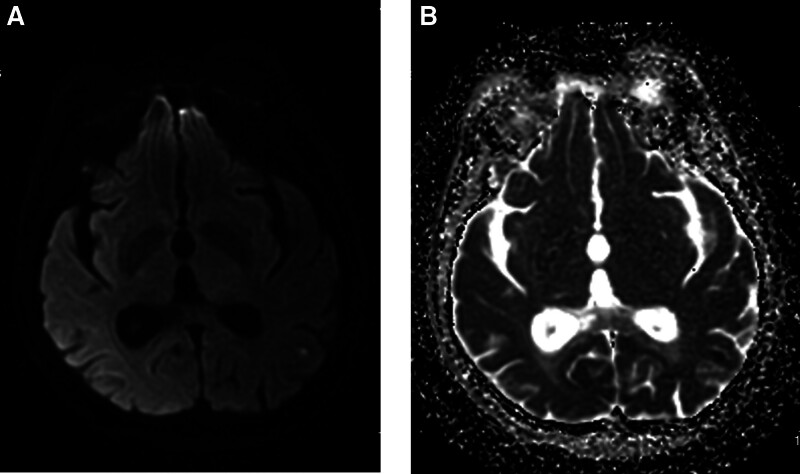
Follow-up cranial MRI after CAS. (A) Magnetic resonance DWI imaging after CAS, DWI point-like high signal in the left parietal lobe, with possible acute/subacute cerebral infarction. (B) Magnetic resonance ADC imaging after CAS. ADC = apparent diffusion coefficient, CAS = carotid artery stenting, DWI = diffusion-weighted imaging.
